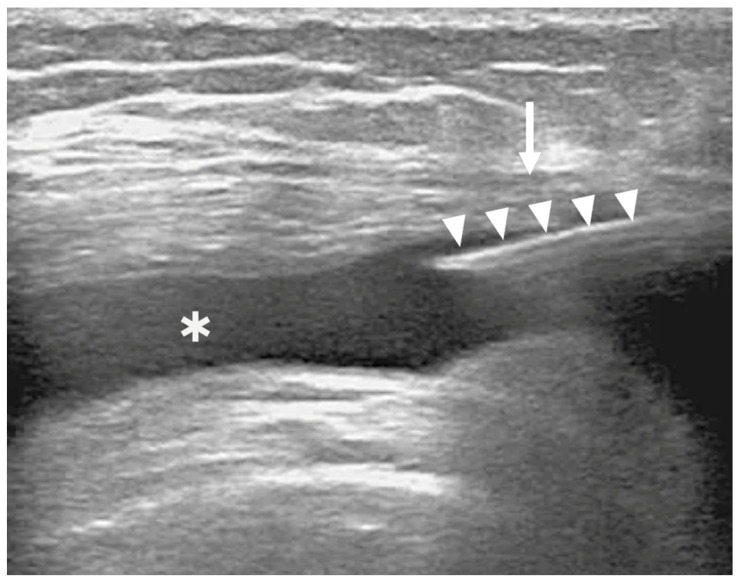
Ultrasound image showing intra-articular BMC injection in the knee. The arrowheads indicate the needle; the asterisk marks the suprapatellar pouch; the arrow points to the medial patellofemoral ligament.
